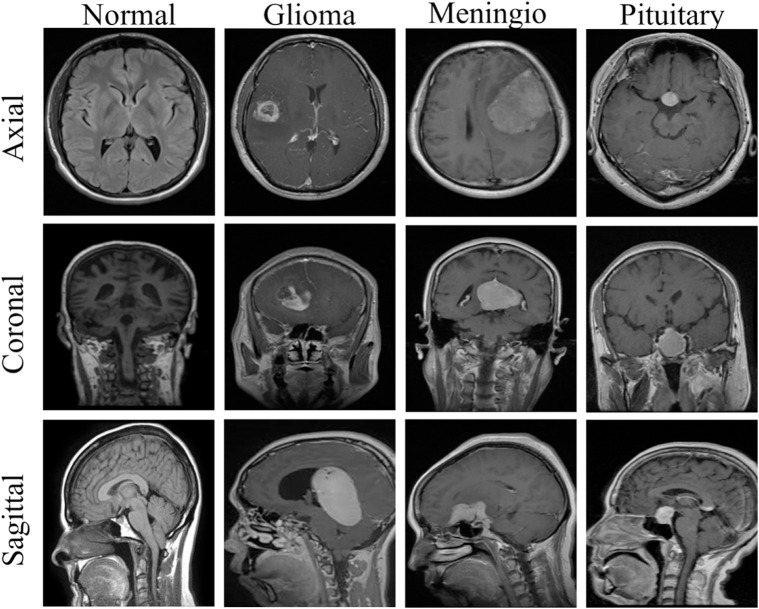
Different views and types of Brain MRI [8].
